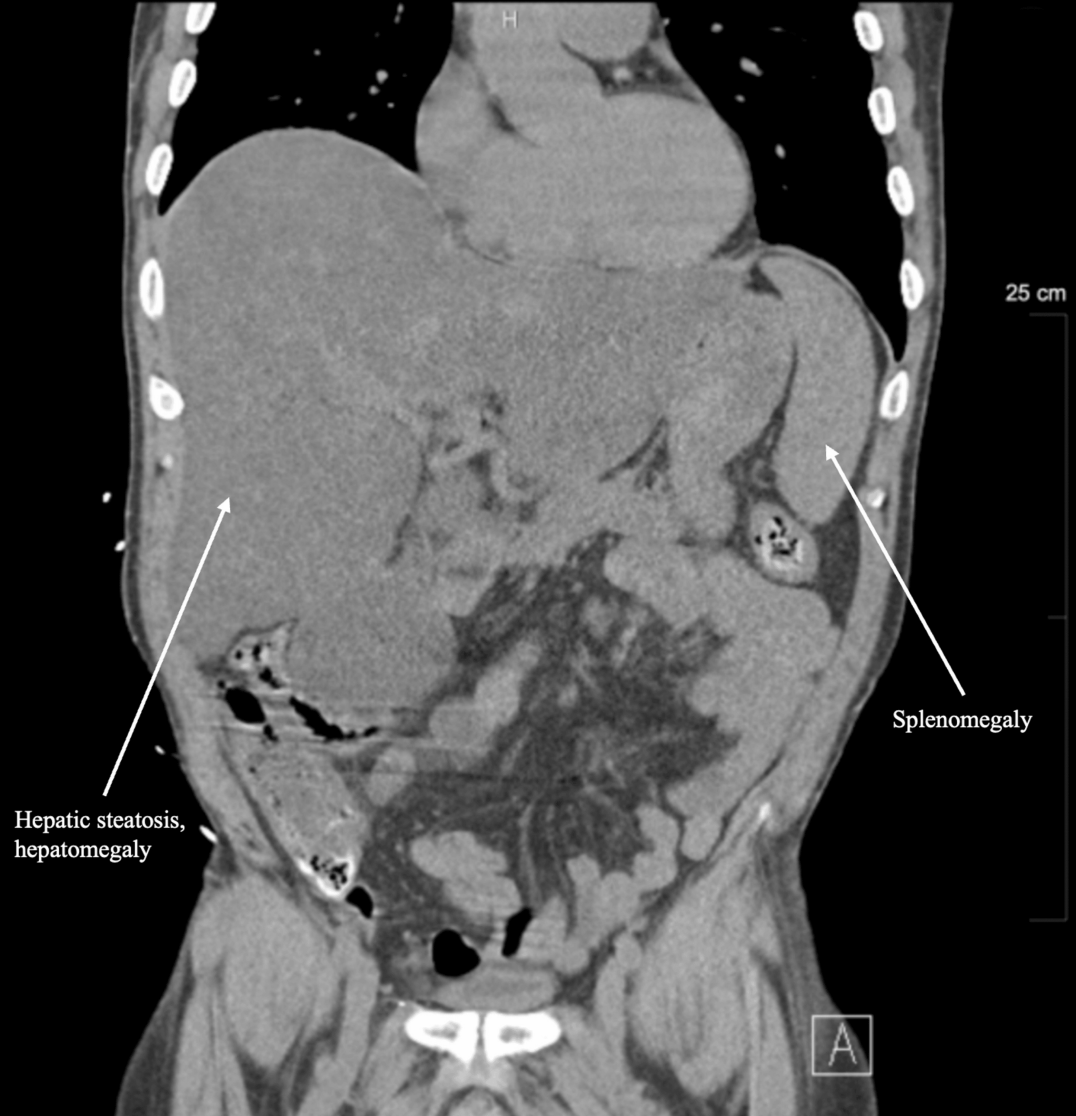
Non-contrast CT scan of the abdomen and pelvis (hospital day one). Initial non-contrast CT of the abdomen and pelvis demonstrating hepatic steatosis, hepatomegaly, distended gallbladder, and splenomegaly.
